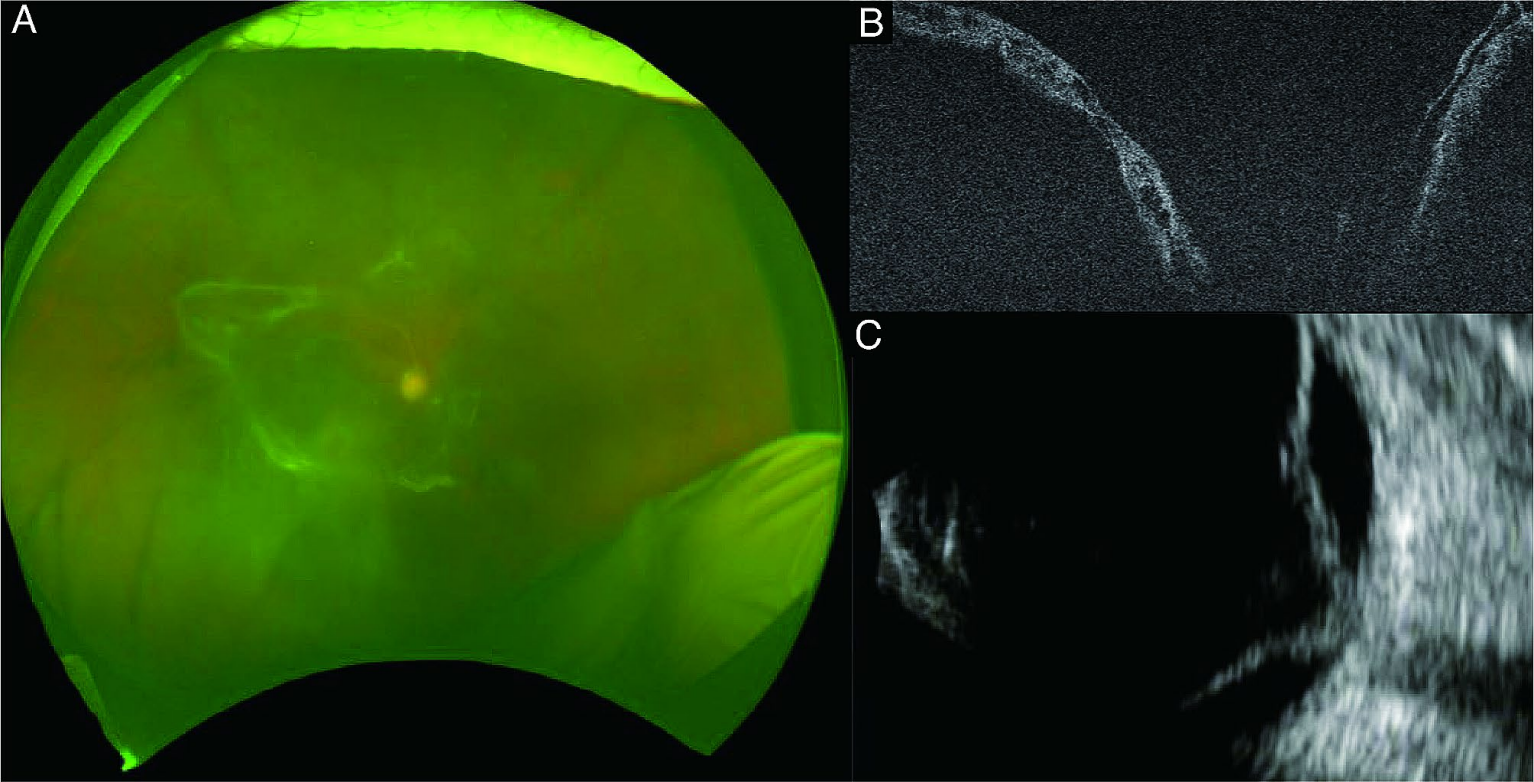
Preoperative images. (A) Retinal detachment and fibrovascular membrane (FVM) were found partially, but it was difficult to investigate the detailed fundus because of the dense cataract. (B) Optical coherence tomography showed the macular retinal detachment and FVM. (C) B-scan ultrasonography detected possible retinal detachment associated with FVM
